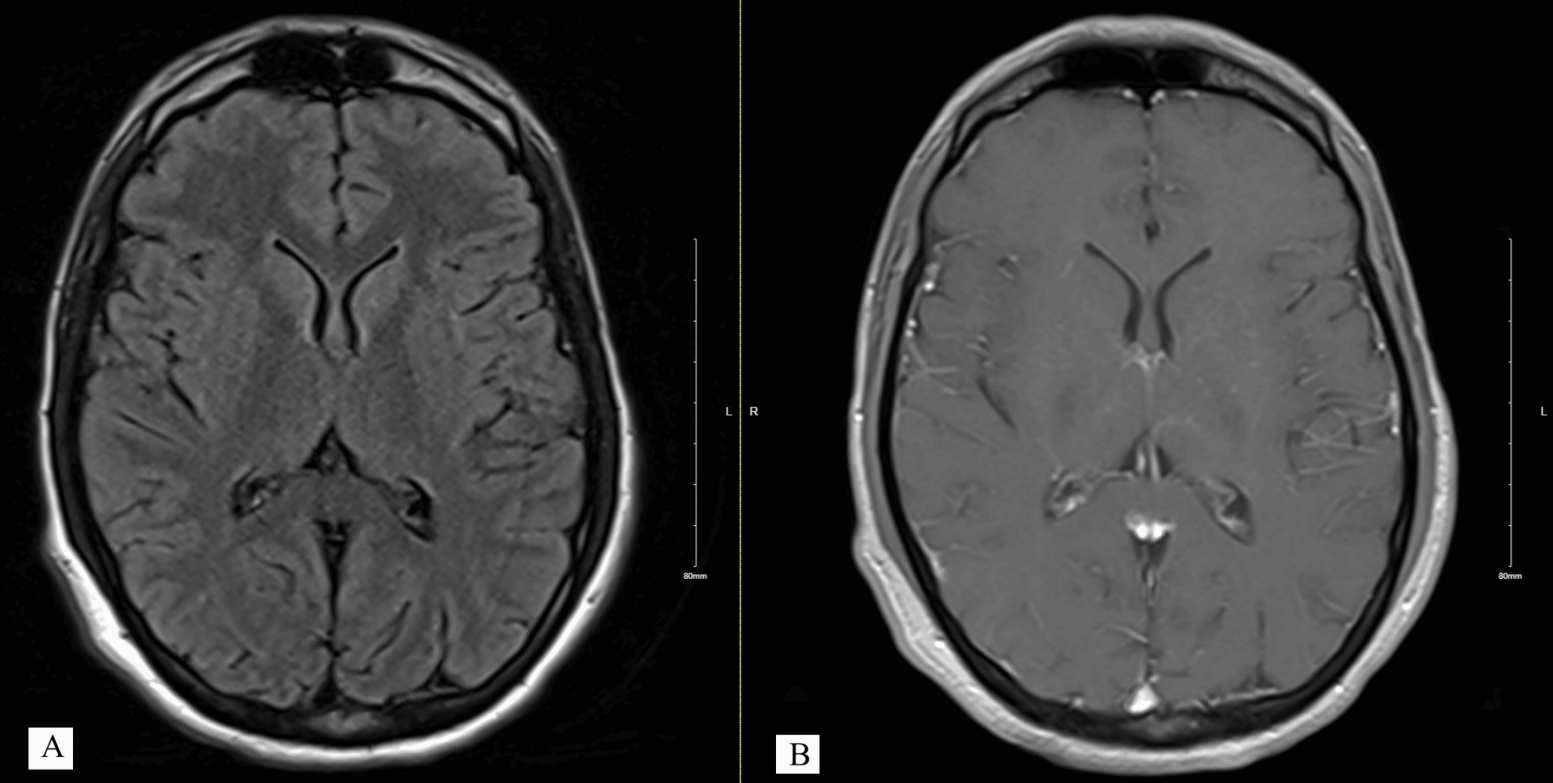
Initial MRI brain within normal limit. A. T2 FLAIR MRI brain on the left, B. T1 Post contrast MRI brain without abnormal contrast enhancement FLAIR: Fluid-attenuated inversion recovery.
